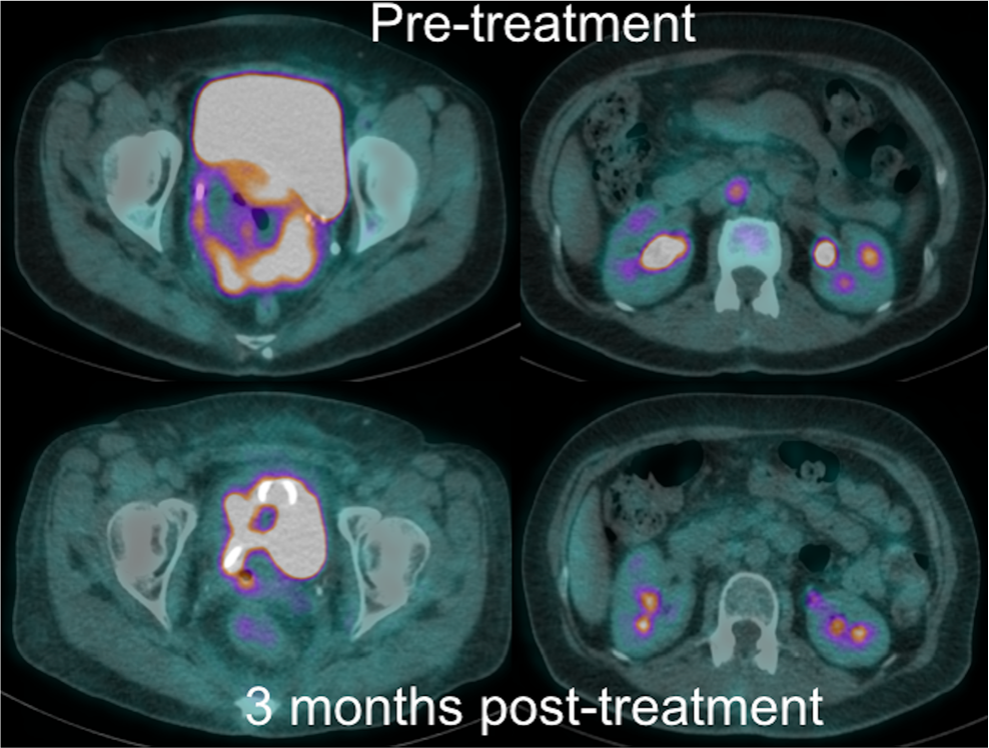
PET-CT imaging performed at baseline (top row) and 3 months post-CRT (bottom row) in a 46-year-old patient with stage 4A disease shows a bulky primary tumour with distal right ureteric involvement (top left) and isolated aortocaval nodal disease (top right). Following treatment there was a good metabolic and morphological response to treatment with only a small residual indeterminate focus of tracer uptake within the cervix (Score 3, bottom left) and minimal activity within the aortocaval node (Score 2, bottom right). The patient relapsed 9 months post-treatment with the development of extensive nodal disease, liver and lung metastases (not shown). She was treated with palliative chemotherapy
